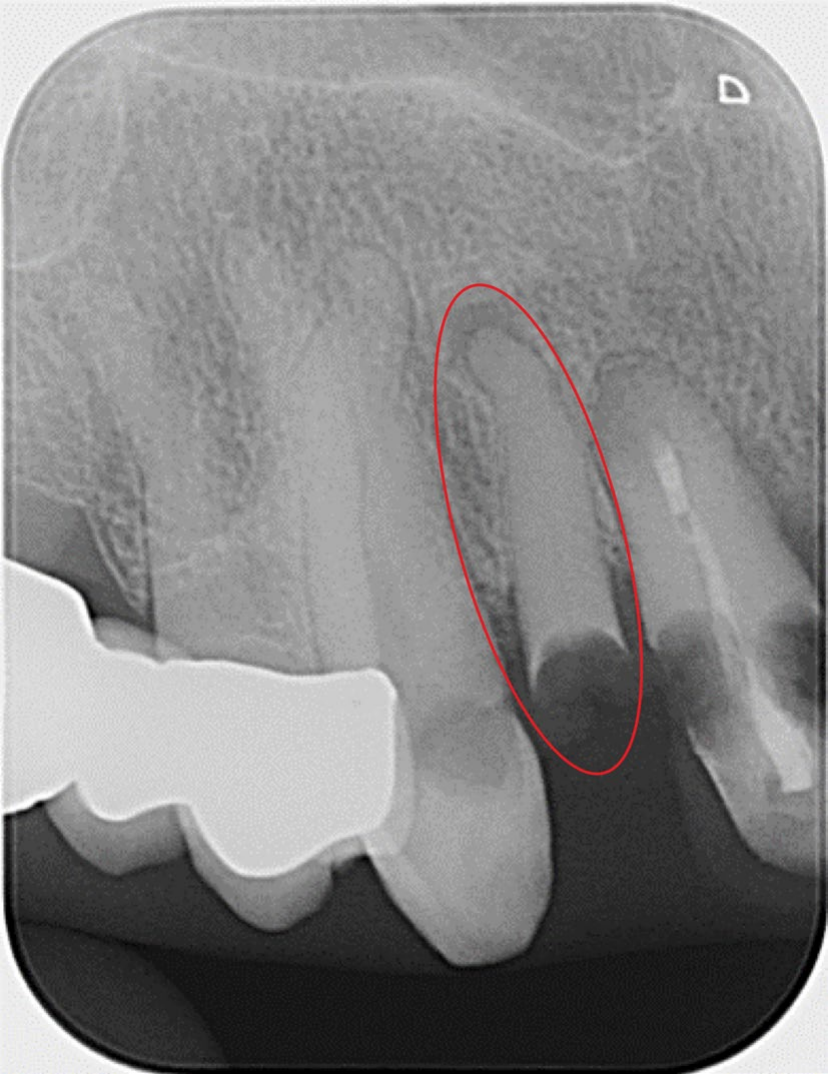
An example of a high‐quality radiograph. In addition, there is a secondary finding (highlighted in red) which, in our opinion, is decisive for the dental diagnosis and treatment. Findings: Tooth 11 with insufficient root canal filling and extensive caries on the mesial and distal aspect of the tooth. Caries on mesial side of tooth 13. Clinically invisible, secondary finding of a root remnant of tooth 12 with apical periodontitis. [Colour figure can be viewed at wileyonlinelibrary.com]
